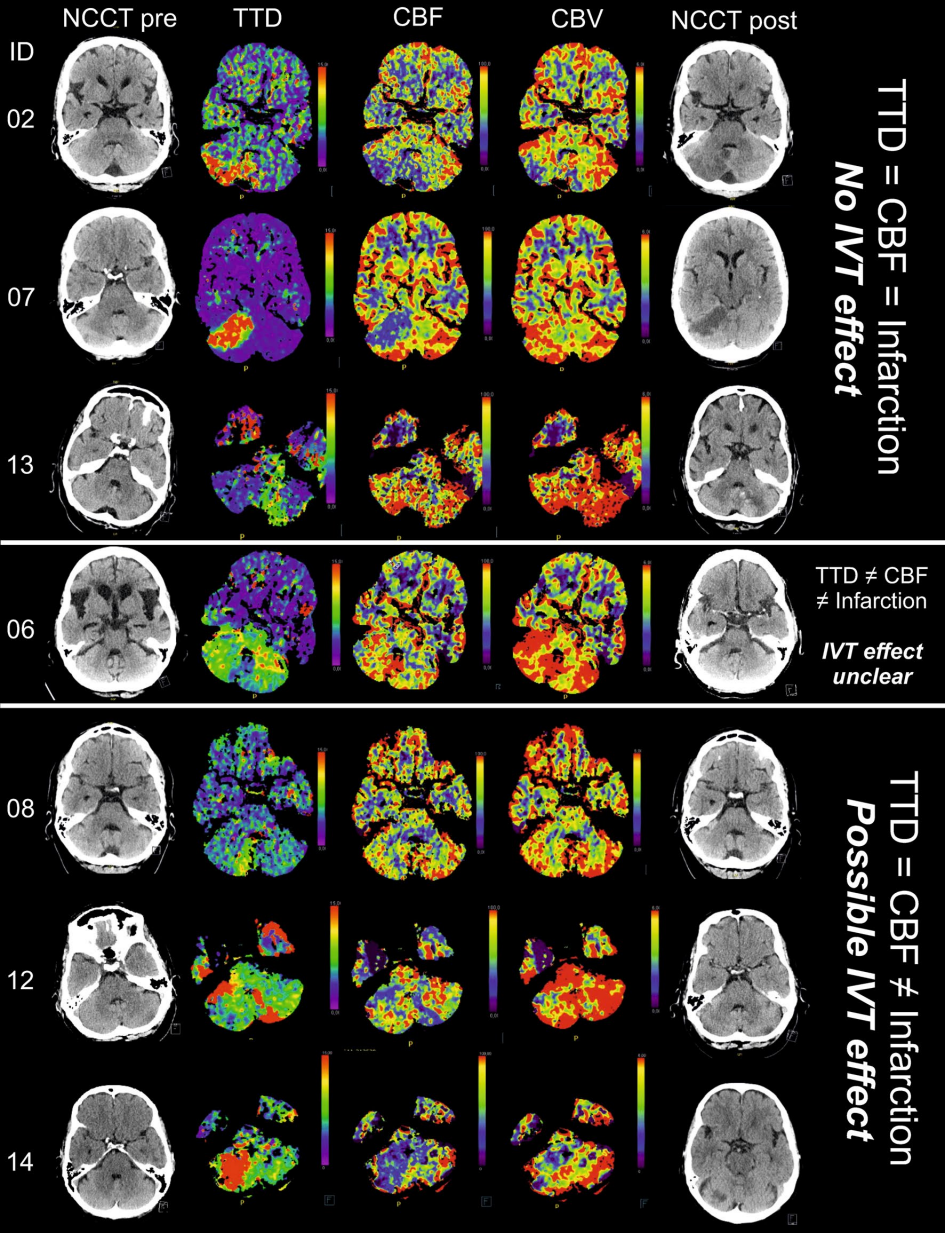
The effect of intravenous thrombolysis (IVT) with regards to imaging parameters: non-contrast CT (NCCT) and CT perfusion (CTP) as well as follow-up NCCT for IVT-treated patients with CTP abnormalities. Despite IVT treatment, three patients (ID 02, 07, 13) finally revealed stroke lesions on follow-up NCCT that matched the areas of hypoperfusion on initial CTP [prolongation of the time-to-drain (TTD) and reduction of cerebral blood flow (CBF)]. Hence, IVT seemed to have no effect in these patients, at least with regards to CT imaging parameters. One patient (ID 06) initially revealed a TTD prolongation but normal CBF in areas of the posterior circulation and there was no infarction on follow-up NCCT. The IVT effect here is unclear. The remaining three patients (ID 08, 12, 14) may have benefited from IVT with regards to imaging parameters. There was either no stroke lesion on follow-up NCCT (ID 08, 12) or an area of infarction that was smaller (ID 14) than the initial CTP abnormality would have predicted
